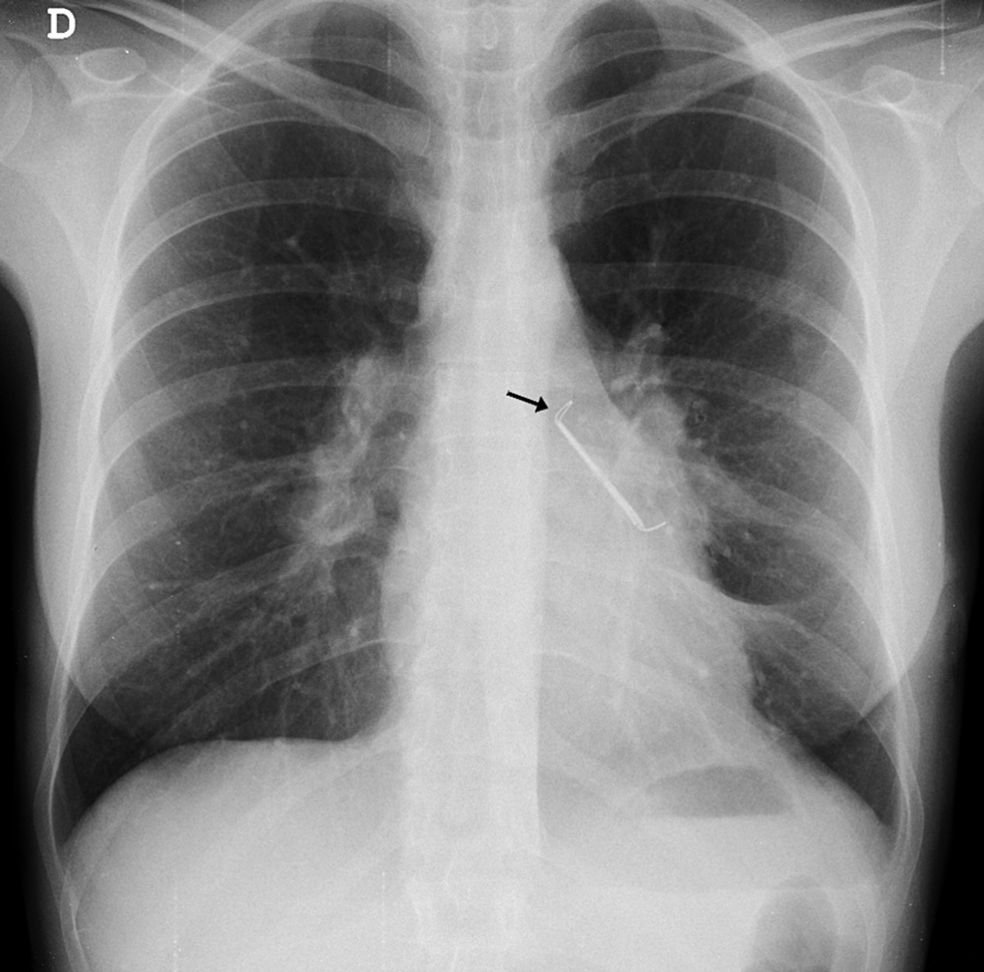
Follow-up chest x-ray the day after surgery The atrial clip (arrow) can be seen in the correct position, and there are no signs of complications.
